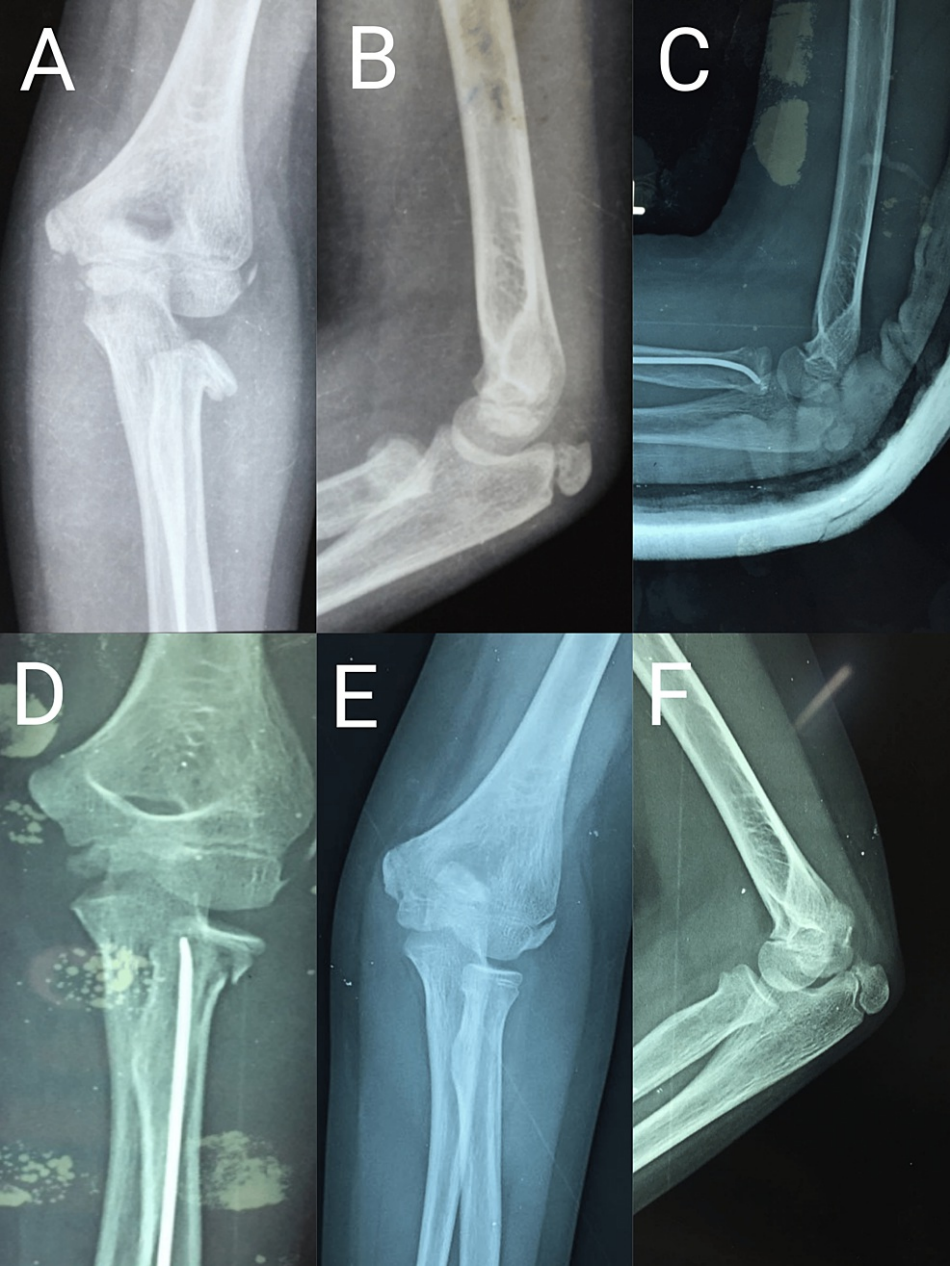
Radiographs of a nine-year-old female patient A and B: Preoperative radiograph of a nine-year-old female patient showing Judet type IV radial neck fracture; C and D: Immediate postoperative radiograph after percutaneous K-wire assisted joystick reduction followed by intramedullary nailing by the Metaizeau technique; E and F: Radiograph at the two-year follow-up showing fracture union
